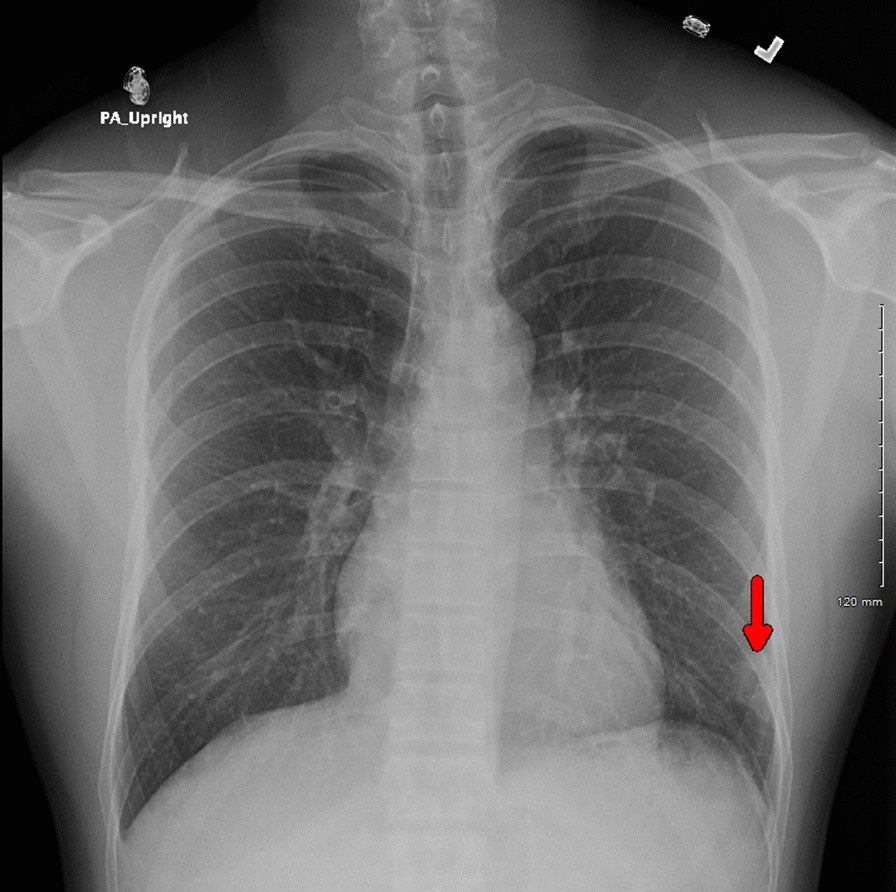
Chest radiography showing a lobular irregularly shaped soft-tissue density at the left base laterally (red arrow)
